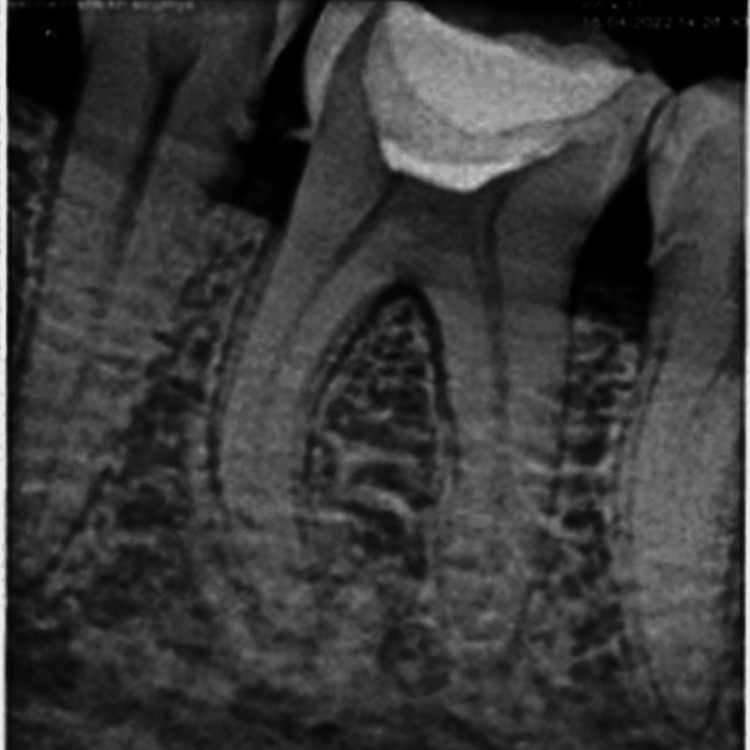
Recall radiograph after two weeks
